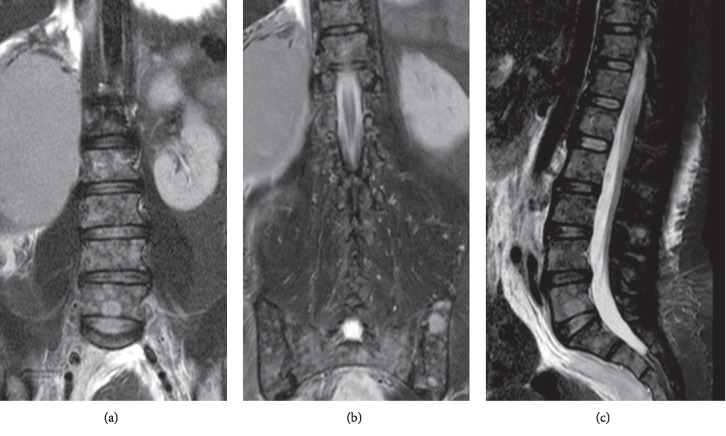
Magnetic resonance images enhanced in short inversion time inversion recovery (STIR) in coronal (left and middle) and sagittal (right). The alteration in the signal of the bone marrow of the vertebral bodies and both iliac bones can be seen by countless discrete images with hyperintense signals of contours, rounded, diameters of up to 13 mm, which are compatible diffuse commitment by multiple myeloma. In addition, the asymmetry in the size of both kidneys can be observed. The adequate attention in a lesion suggestive of neoplasia.
